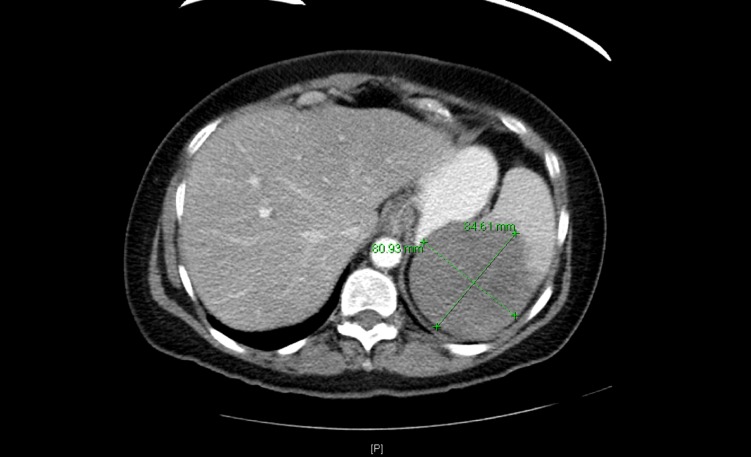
A CT scan of the abdomen in October 2015 A hypodense mass measuring 8.5 x 8.1 x 8.8 cm is seen within the spleen.
